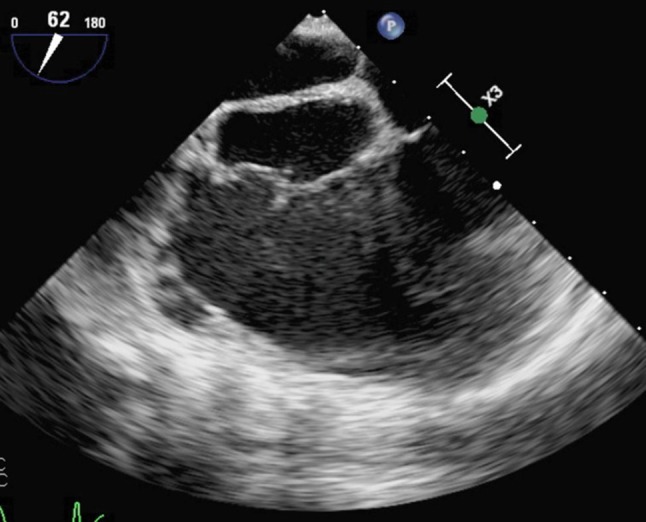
TEE (transoesophageal echocardiography) with a 4 chamber view
